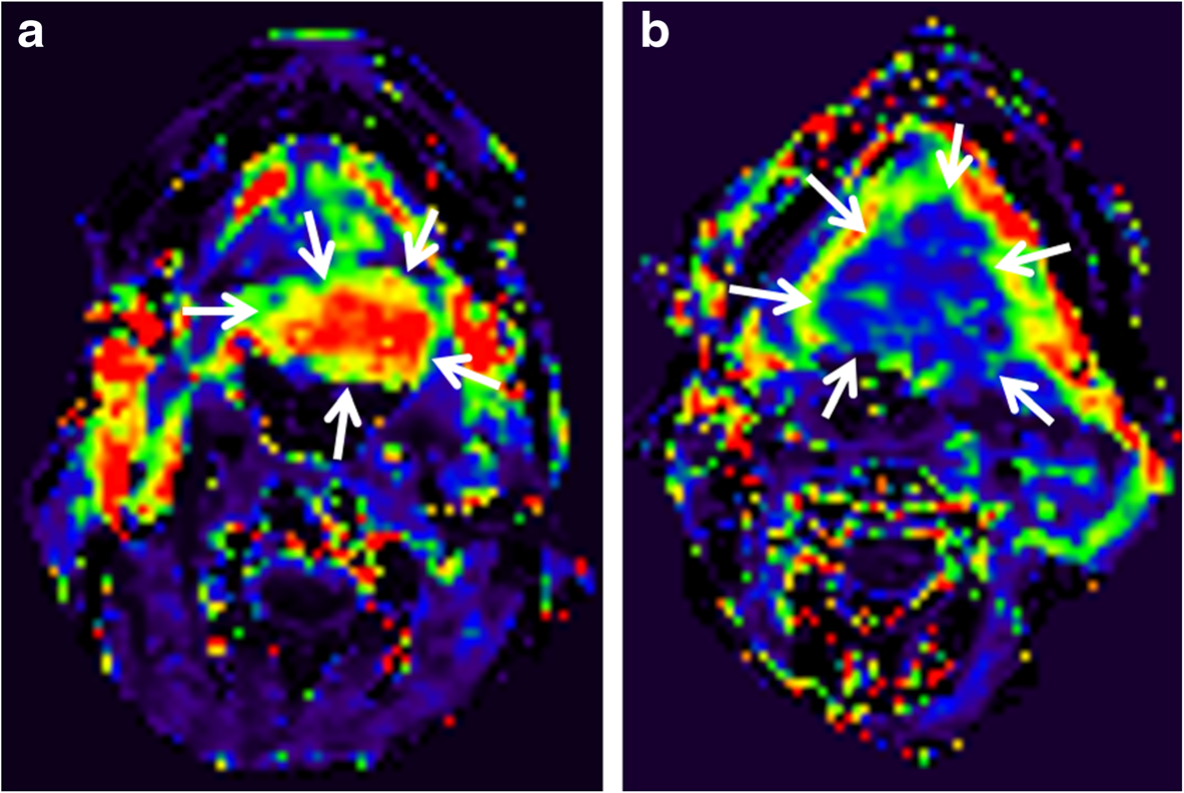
a & b. Pre-treatment DCE-MRI for prediction of CRT response. a Pre-treatment K^trans^ map of a squamous cell carcinoma of the tongue (*arrows*) with a high K^trans^ (0.87 min^−1^) in a tumour with local control; b Pre-treatment K^trans^ map of a squamous cell carcinoma of the tongue (*arrows*) with a low K^trans^ (0.29 min^−1^) in a tumour with local failure
